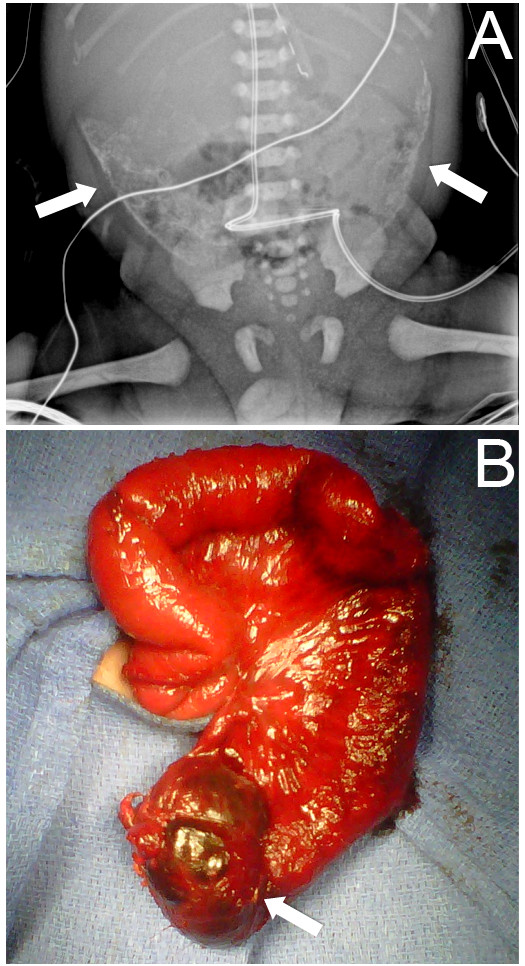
Initial radiographic image and photograph taken after patient’s second operation. ( A) Plain abdominal radiograph showing bilateral eggshell calcifications consistent with giant cystic meconium peritonitis (GCMP, arrows). ( B) Photograph taken at the patient’s second operation, where the peritoneal drain was removed and an ileostomy was brought out. The remnant of the original meconium pseudocyst can be seen at the bottom of the figure (arrow).
